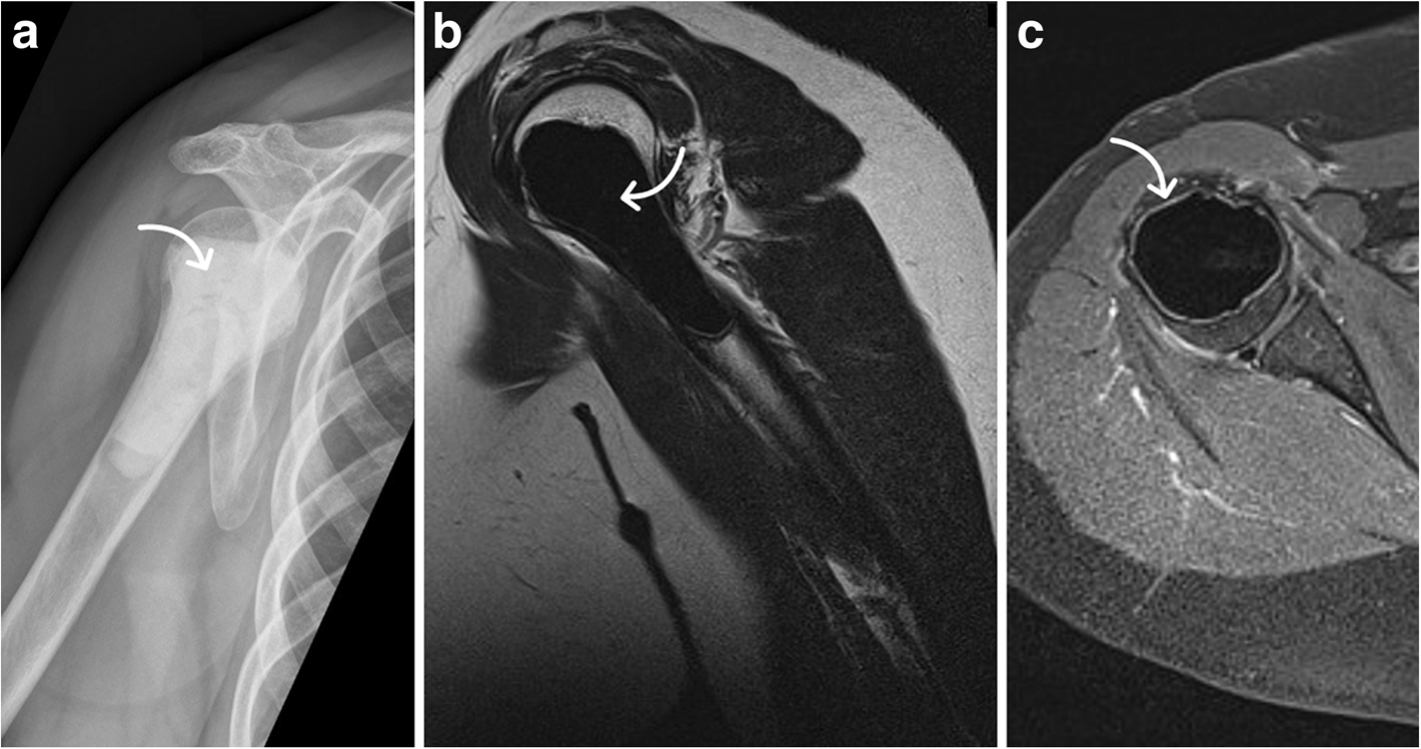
Bone cement filling without osteosynthesis after rigorous intralesional tumor resection. The large bone cement filling (arrow) can be easily depicted in plain radiographs (a) and MRI with T2-weighted sagittal series (b) and T1-weighted contrast-enhanced axial series (c). Although filling out the complete proximal humerus, sufficient stability was achieved without additional osteosynthesis as no postoperative fractures were found later on for all equally treated cases. Typical edema line (arrow in c) after bone cement implantation must be distinguished from local recurrence
